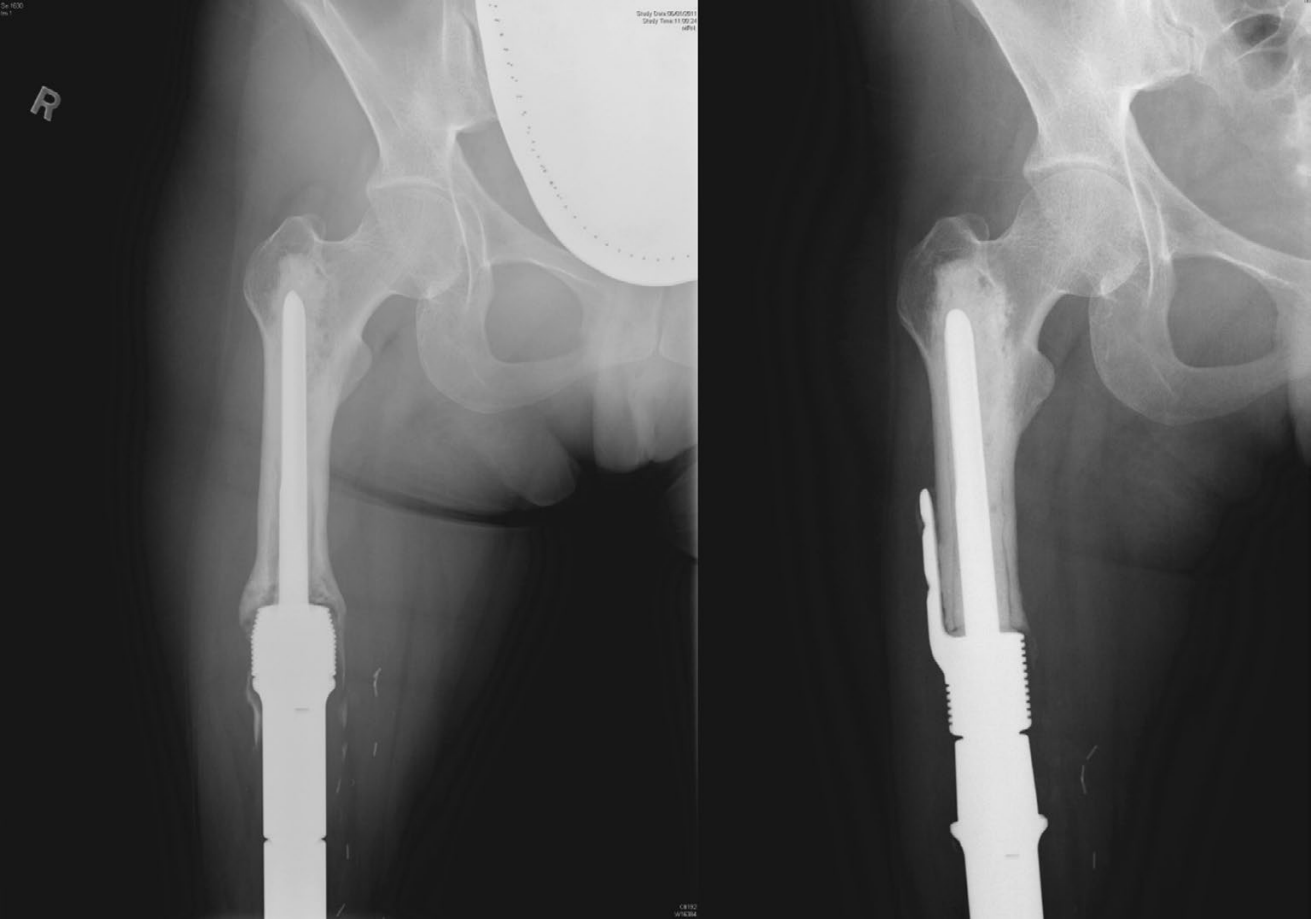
Left: Plain radiograph of aseptic loosening of a previous distal femur endoprosthesis following failed allograft reconstruction for the treatment of osteosarcoma. Infectious workup was negative. Right: Immediate postoperative plain radiograph of the revision to a distal femur endoprosthesis with HA‐coated stem collar and an extracortical plate.
